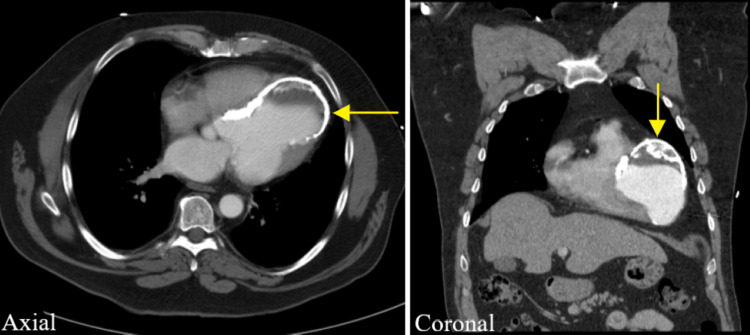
Axial and coronal intravenous contrasted CT images showing the calcified border of left ventricular aneurysm (yellow arrows). A partially calcified thrombus is also seen within the aneurysm.
